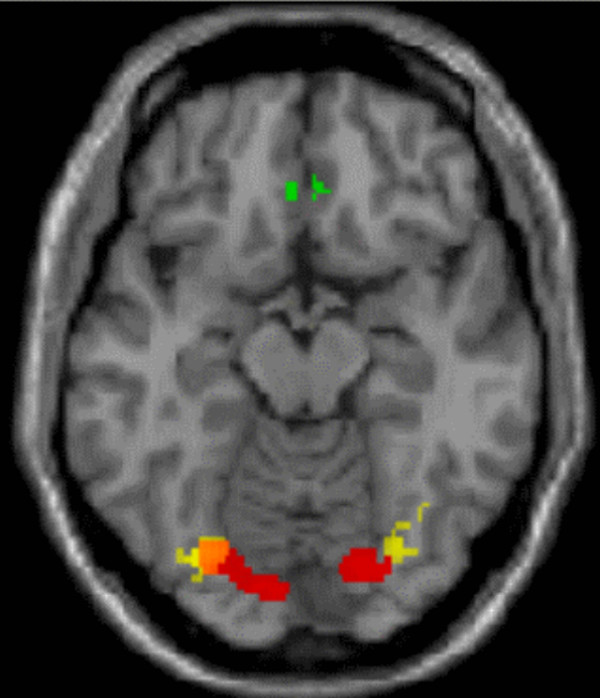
Brain areas involved in PC1 and PC2 superimposed on the canonical single-subject MR image of SPM2 in an axial plane showing the lateral position of activity in the fusiform face area (PC2, yellow, positive loading) relative to PC1 (red, negative loading). Note the medial prefrontal involvement in PC1 (green, positive loading).
